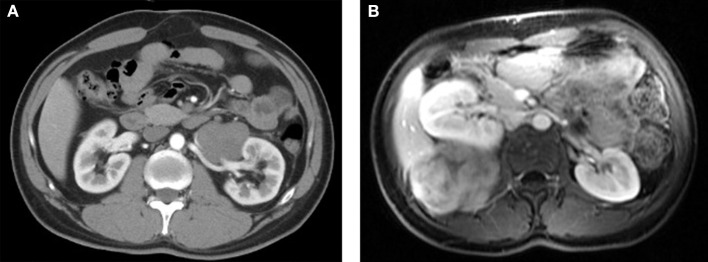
(A) Computed tomography revealed a low-density mass around the left renal artery. (B) Magnetic resonance imaging revealed an isointense mass with a hyperintense signal at the right lumbar psoas muscle.
